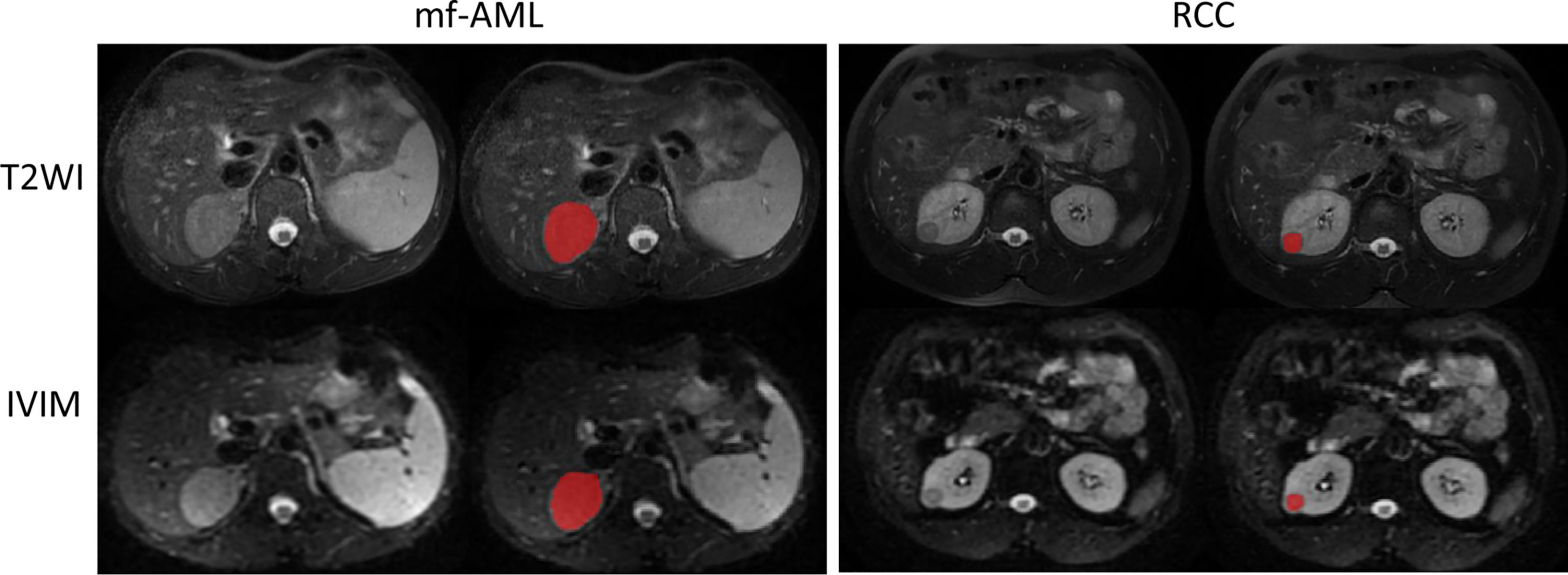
Example of tumor segmented by the radiologist. The mf-AML and RCC were segmented on the T2-weighted image but the same segmentation has been copied to IVIM-DWI (b = 1000 s/m^2^) image.
